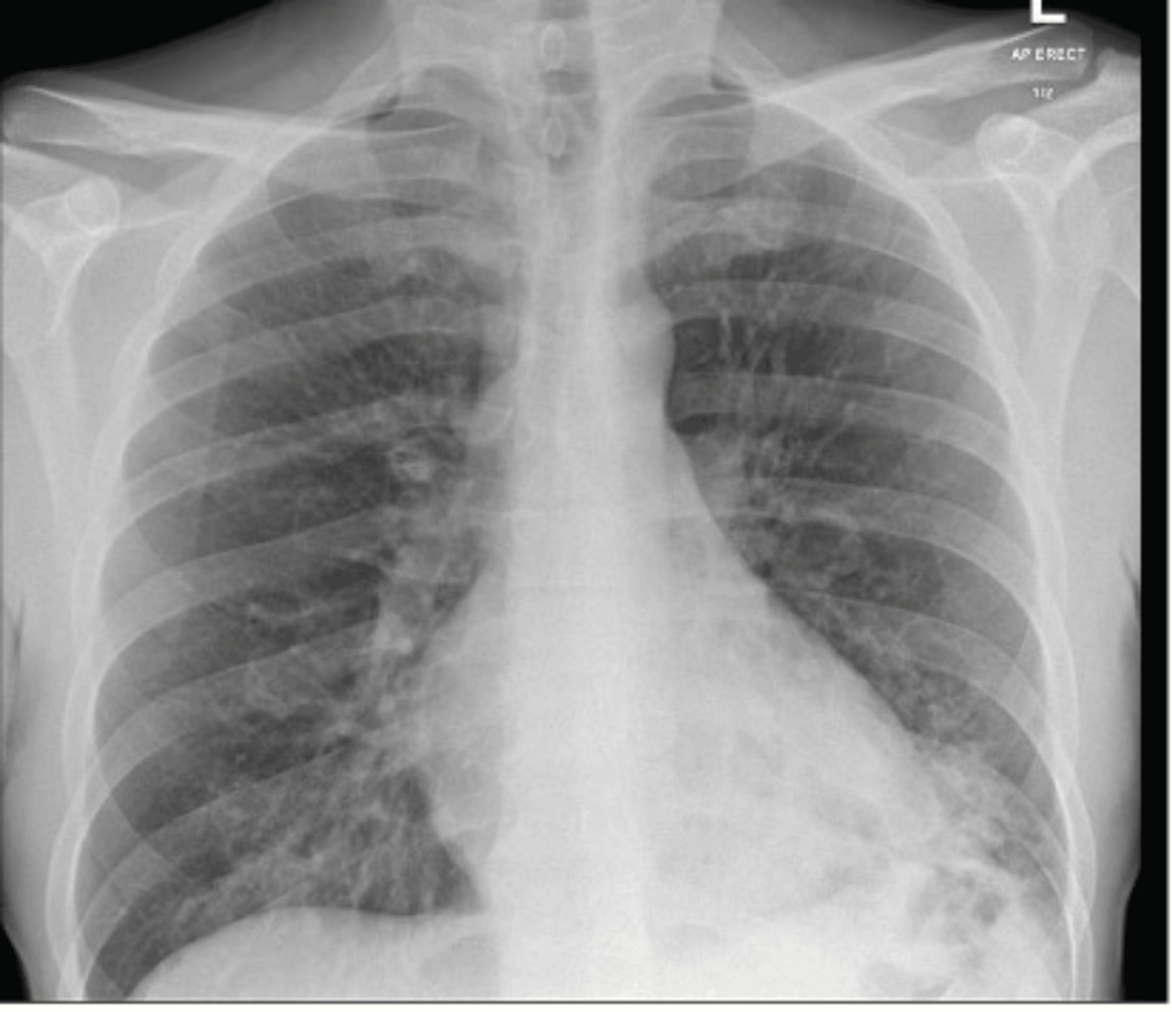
Chest radiograph at initial presentation showing left lower lobe consolidation
